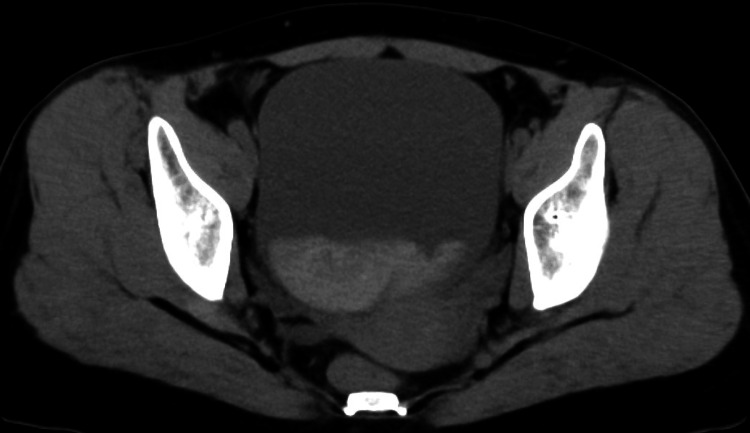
Computed tomography The initial contrast-enhanced computed tomography showed a distended urinary bladder containing hyperdense fluid, indicating clot retention.
